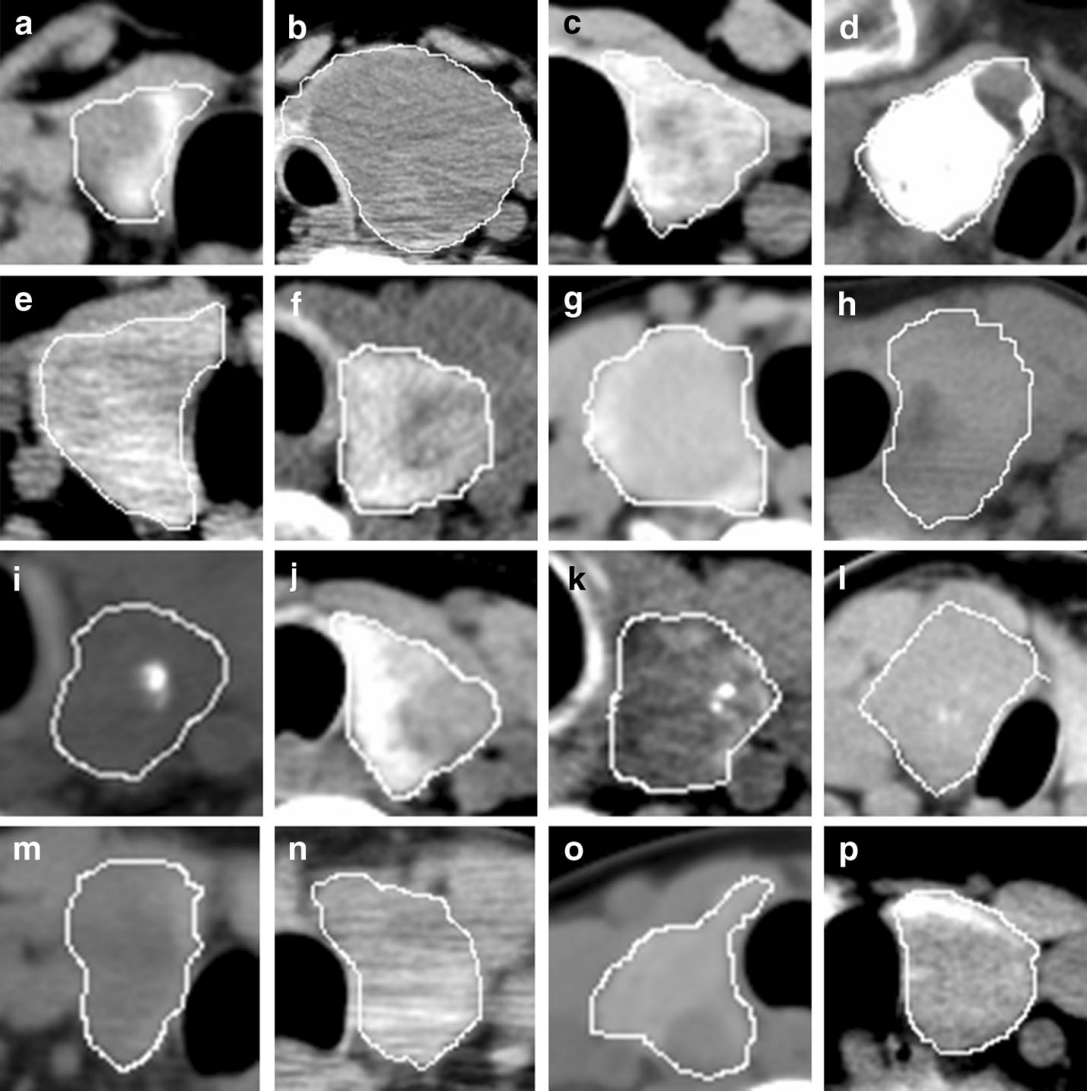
CT images of thyroid nodules from different patients with marked ROIs. Thyroid nodules in images (a–h) are benign. Nodules in images (i–p) are malignant
